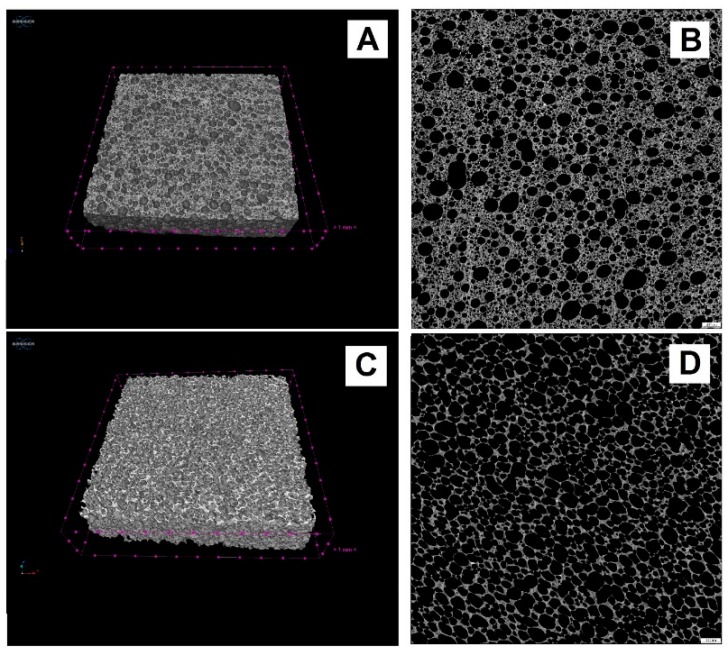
X-ray microtomography images of 3D slice (on the left) and 2D slice (on the right) of the GSil03 sample (A,B) and of the GSil12 sample (C,D). The scale bar is 500 μm.
